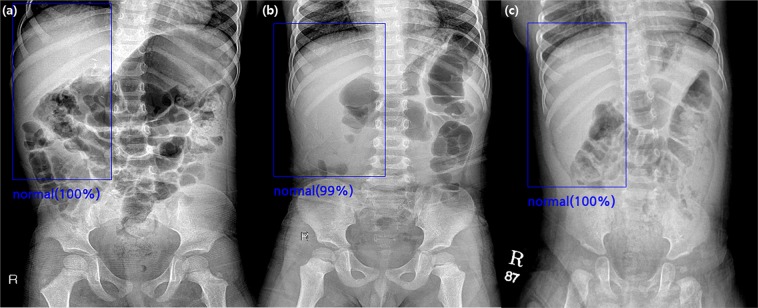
True-negative cases found by the algorithm in the validation set. (a) A supine abdominal radiograph of a 36-month-old girl without intussusception. All four radiologists and the algorithm reported true-negative results because there was no round opacity obscuring bowel gas in the right abdomen. (b) A supine abdominal radiograph of a 48-month-old boy without intussusception. Two radiologists reported false-positive results, while the algorithm and the other two radiologists reported true-negative results. (c) A supine abdominal radiograph of an 11-month-old boy without intussusception. Four radiologists and the algorithm all reported true-negative results.
